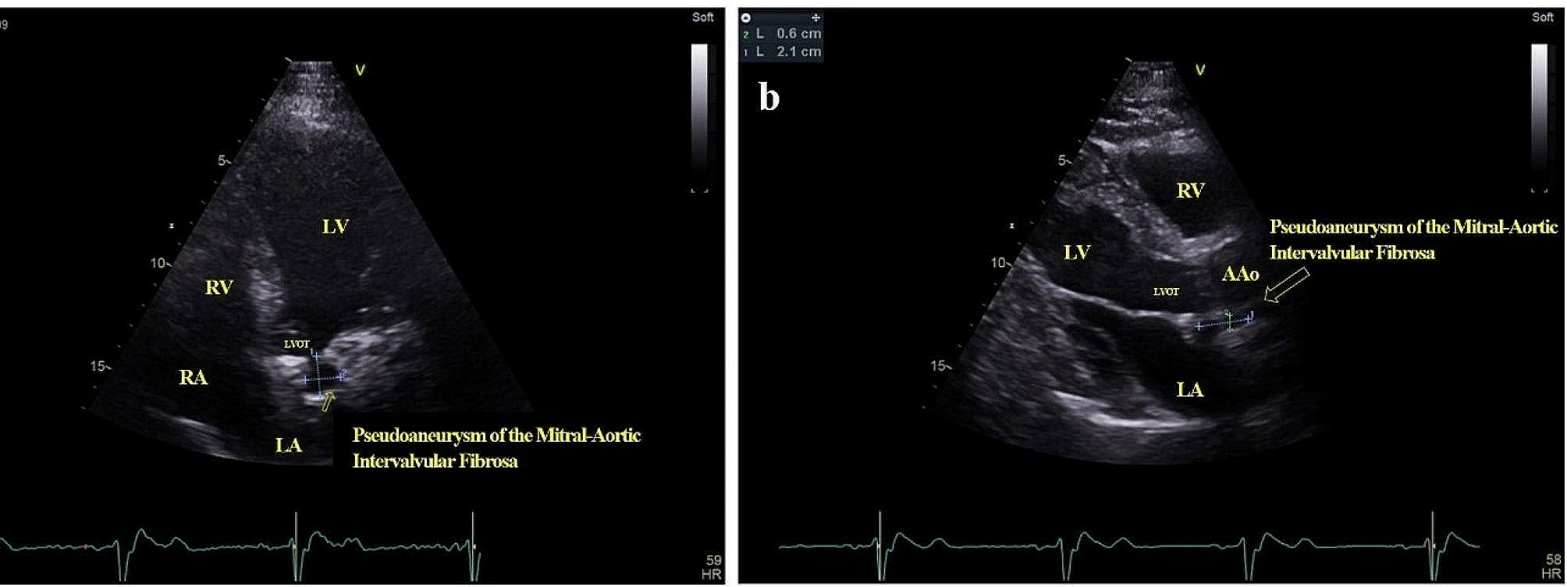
Transthoracic echocardiography revealed PMAIF: (a) four-chamber view of a PMAIF (arrow), size = 1.5 × 1.7 cm; (b) parasternal long-axis view of a PMAIF (arrow), size = 0.6 × 2.1 cm LV: left ventricle, RV: right ventricle, RA: right atrium, LA: left atrium, LVOT: left ventricular outflow tract, AAo: ascending aorta
